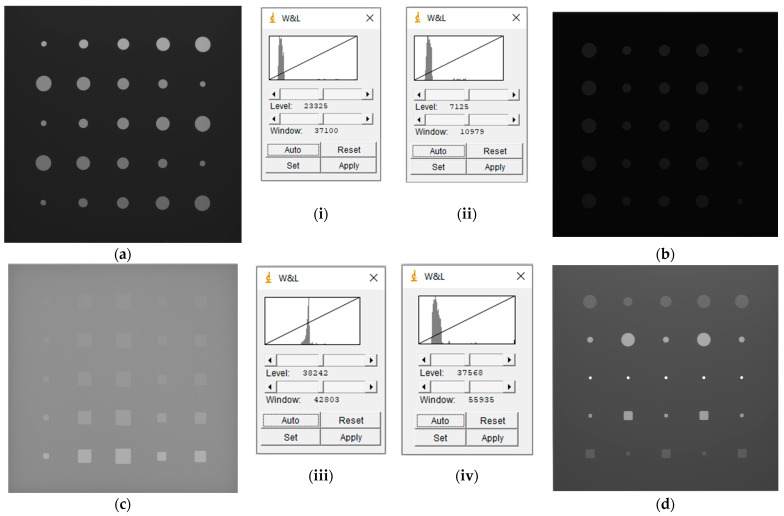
A cross-section of radiographic images of plates captured at different exposure factors: (a) 60 kV_200 µA_0.5 s, (b) 70 kV_100 µA_0.2 s, (c) 150 kV_50 µA_0.5 s, and (d) 60 kV_400 µA_0.5 s. All 16-bit images show standard gray values, with no post-acquisition image enhancement. Using ImageJ version 1.53t [48], the window and level displayed in images (i–iv) represent (a–d), respectively.
